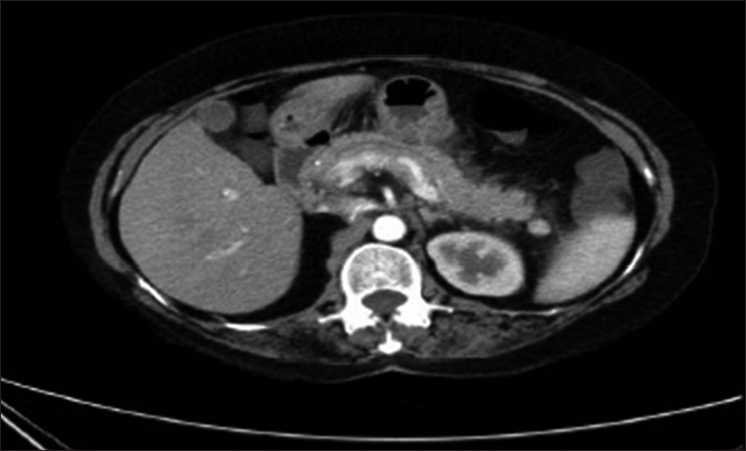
Contrast-enhanced computed tomography of pancreas showing peripancreatic fat standing
